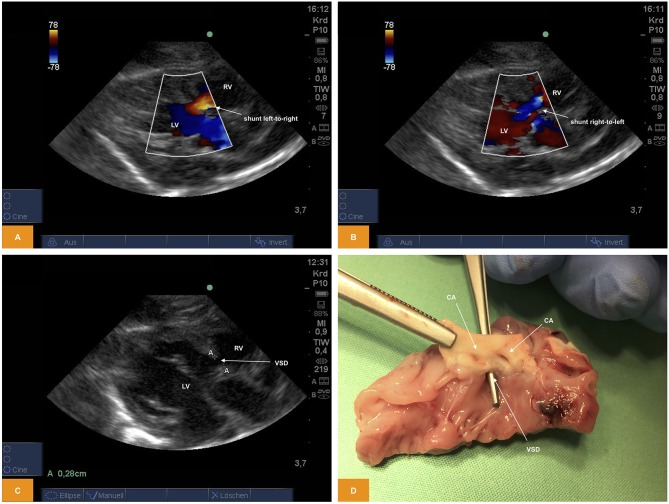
(A,B) Color Doppler image of shunting across a ventricular septal defect. The image was obtained from PLAX. Note the bidirectional direction of shunt with red representing left-to-right shunt and blue representing right-to-left shunt (age: 36 h, weight: 1.48 kg). (C) 2D image of a ventricular septal defect from PSAX (age: 36 h, weight: 1.48 kg). (D) Anatomic view of a ventricular septal defect (age: 36 h, weight: 1.48 kg). CA, coronary arteries; LV, left ventricle; PLAX, parasternal long axis; PSAX, parasternal short axis; RV, right ventricle; VSD, ventricular septal defect.
